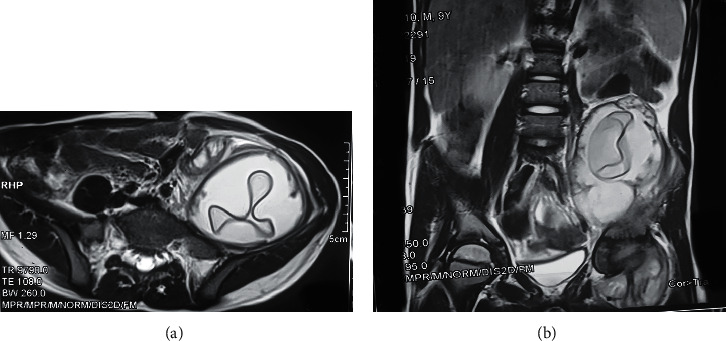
MRI image in transversal (a) and sagittal (b) plane showing a floating membrane of the hydatid cyst.
